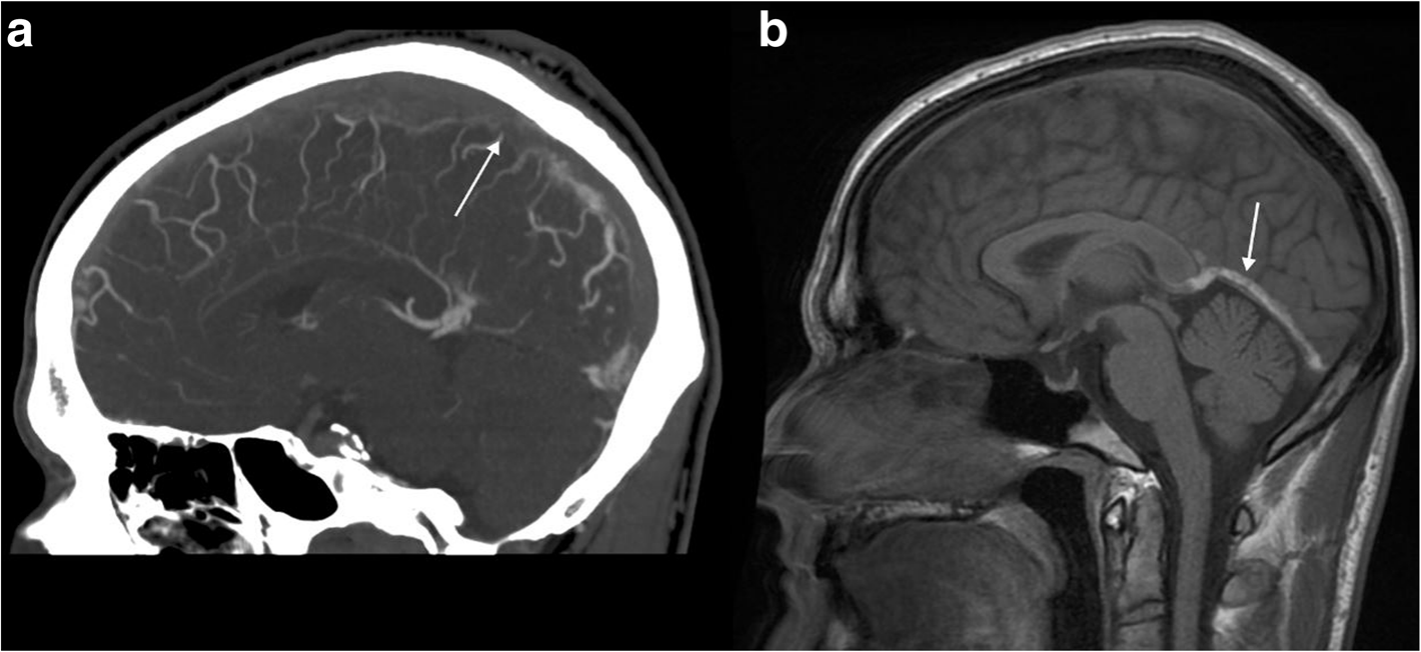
Computed tomography angiography (a) showing an extended superior sagittal sinus thrombosis (arrow) and T1 weighted sagittal magnetic resonance imaging (b) showing thrombosis of the straight sinus (arrow).
